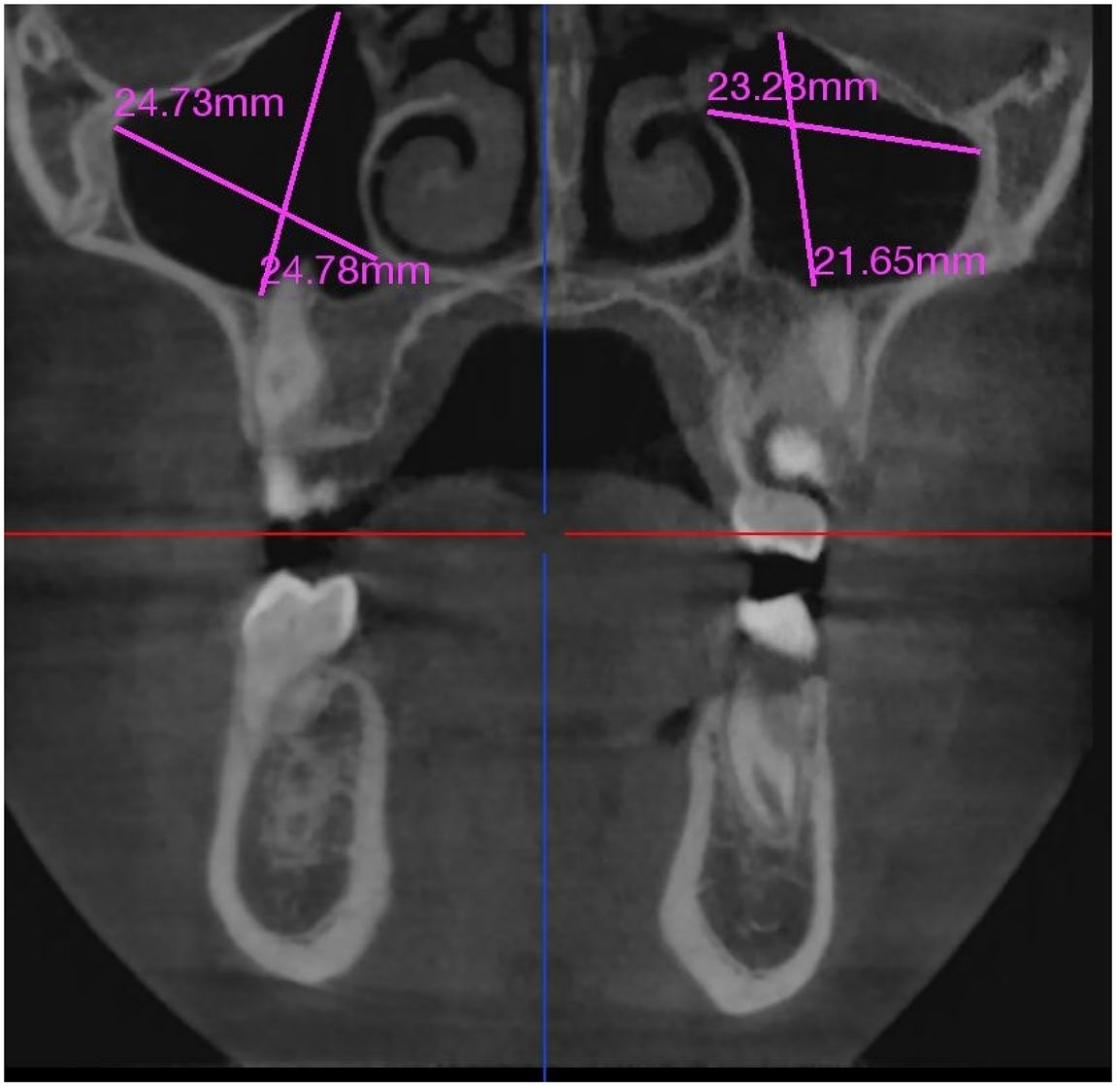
Coronal CBCT view demonstrating linear measurements of the maxillary sinuses.
